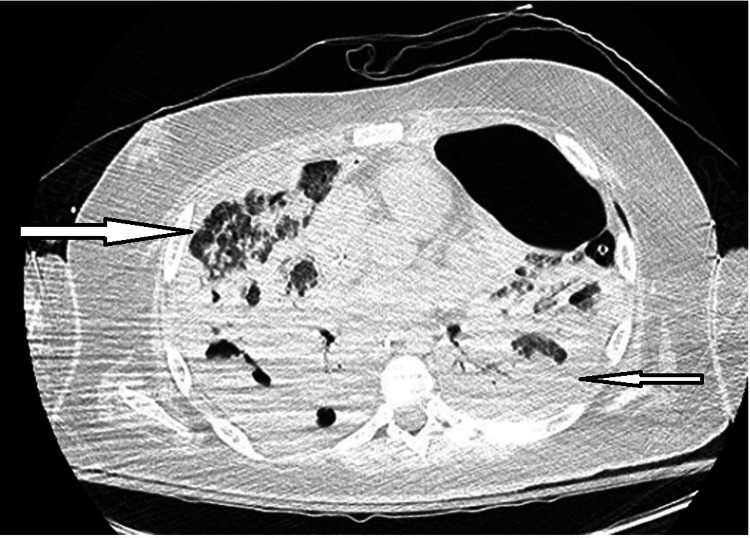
CT scan of the chest demonstrating diffuse ground-glass opacities in the upper lung fields and near-complete opacification of the lower lobes bilaterally with multifocal areas of cystic necrosis and cavitary lesions.
